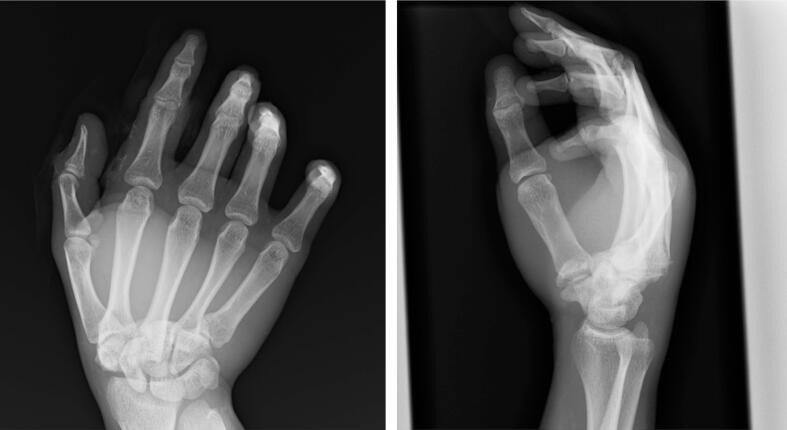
X-rays show dorsal dislocation of the third and fourth CMC joints and loss of the normal articulation of the STT joint.
